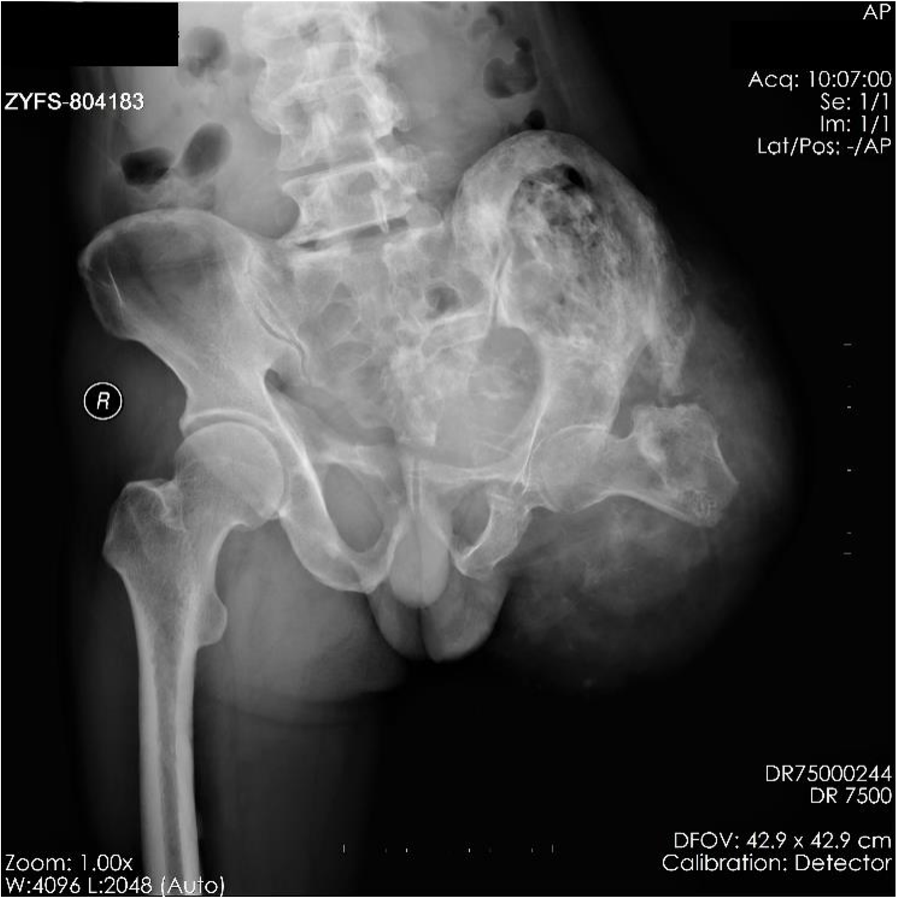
Postoperative X-ray showed fracture site after amputation
